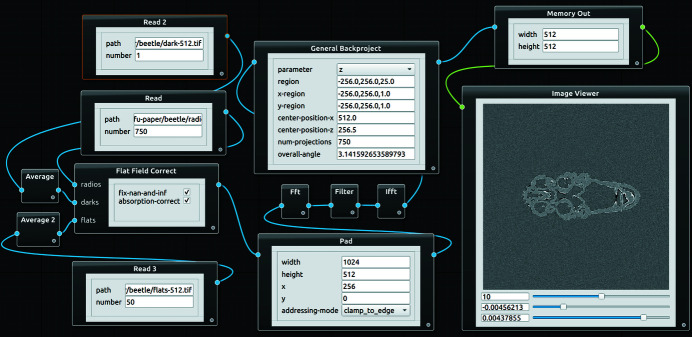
Main window of *tofu flow* showing tomographic reconstruction. Dark and flat field images are averaged and used to flat-field-correct the radiographs. The normalized images are padded in order to remove convolution outlier artifacts, Ram-Lak-filtered in the Fourier space, back projected and displayed.
